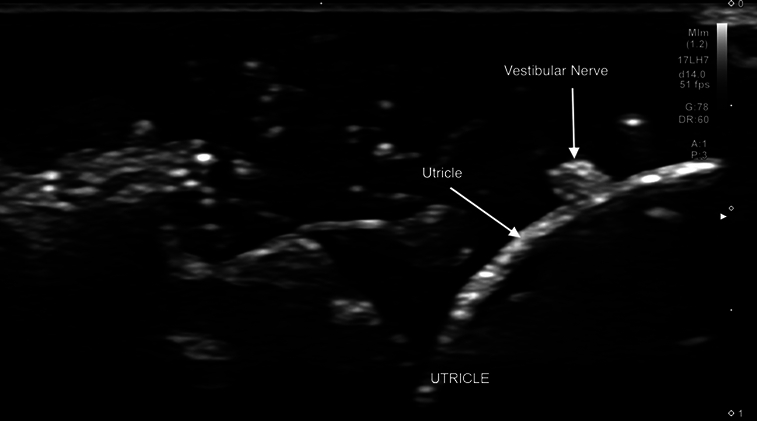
Sonogram (17 MHz) demonstrating the border of the utricle and a short-axis view of the vestibular nerve. The otoliths are deep to the utricle.
